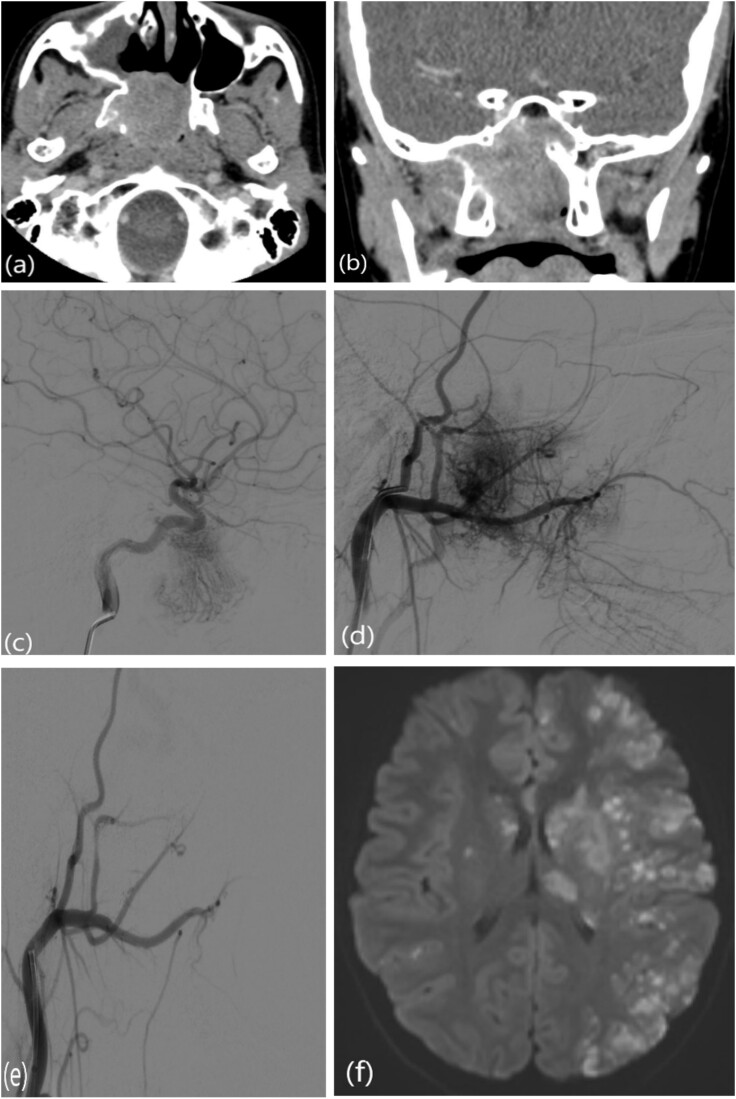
(a, b) Contrast-enhanced CT of the paranasal sinuses showing a right nasopharyngeal mass with extension into the sphenoid sinuses and right pterygoid plate. (c) Lateral projection angiogram of the right ICA demonstrating the blood supply to the right nasopharyngeal mass from the inferolateral trunk. (d) Lateral projection angiogram of the right ECA showing extensive vascular supply to the hypervascular mass from multiple branches. (e) Post-embolization right ECA angiogram showing devascularization of the tumor. (f) Transaxial diffusion-weighted imaging of the brain showing extensive foci of restricted diffusion, predominantly involving the left side, consistent with acute stroke.
